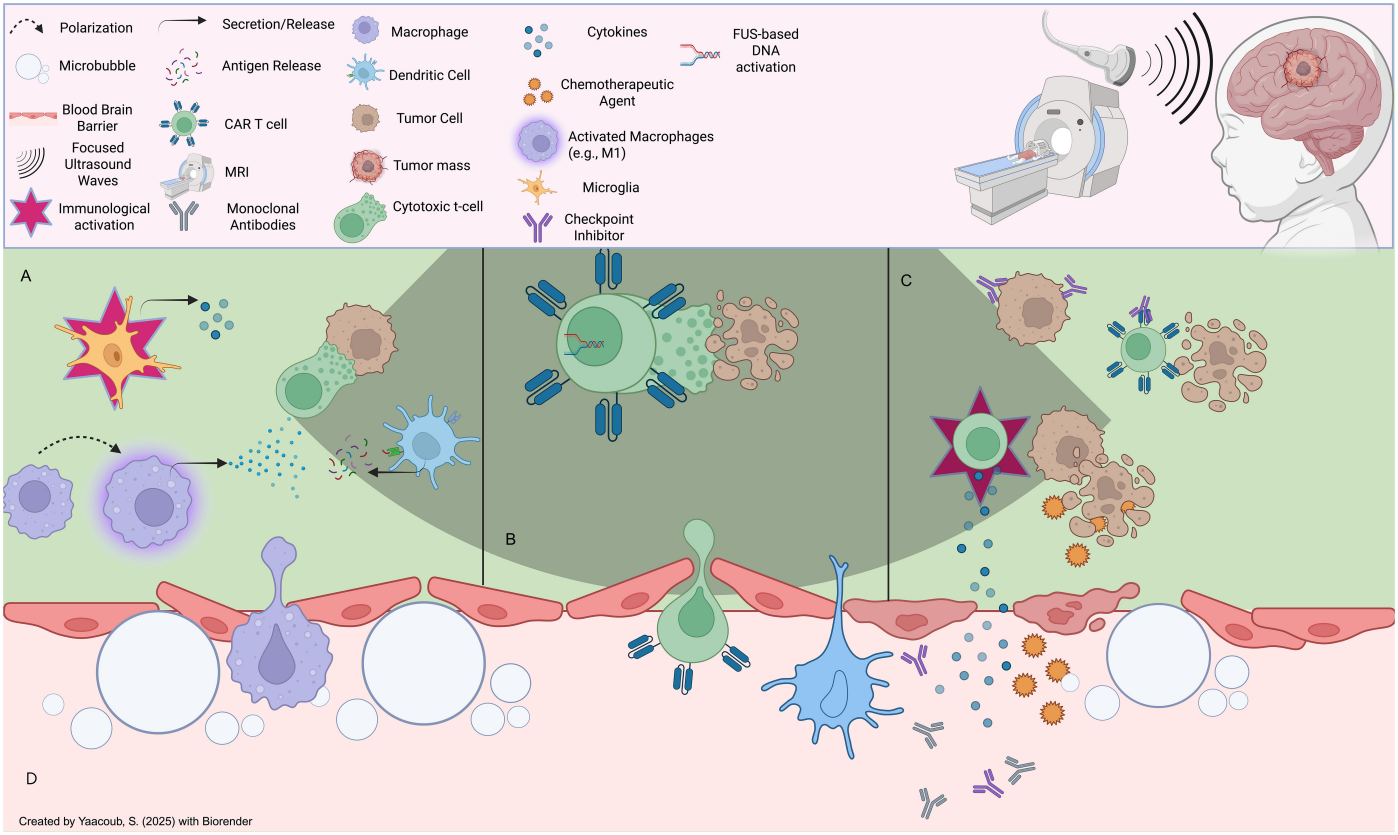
Effects of focused ultrasound on the tumor microenvironment: (A) FUS induces immunomodulatory effects onto immune cells in the TME, such as microglial activation, macrophage repolarization (e.g., M2 to M1), and antigenic presentation of tumor neoantigens onto immune cells (eg., T cells), (B) FUS can be leveraged to induce acousto-genetic, controlled CAR-T cell activation to limit unwanted pro-inflammatory effects (e.g., adverse effects onto host tissues) (C) FUS-mediated BBB opening can mediate CAR-T cell infiltration post-systemic administration intravenously, in addition to entry of other therapeutic agents such as endogenous or extrinsic cytokine and antibody-based therapies that can induce anti-tumor effects. (D) FUS induces reversible BBB disruption through microbubble-based cavitation effects which can loosen tight junctions; this can lead to the infiltration of a plethora of endogenous immune cells as well as extemal therapeutic agents.
